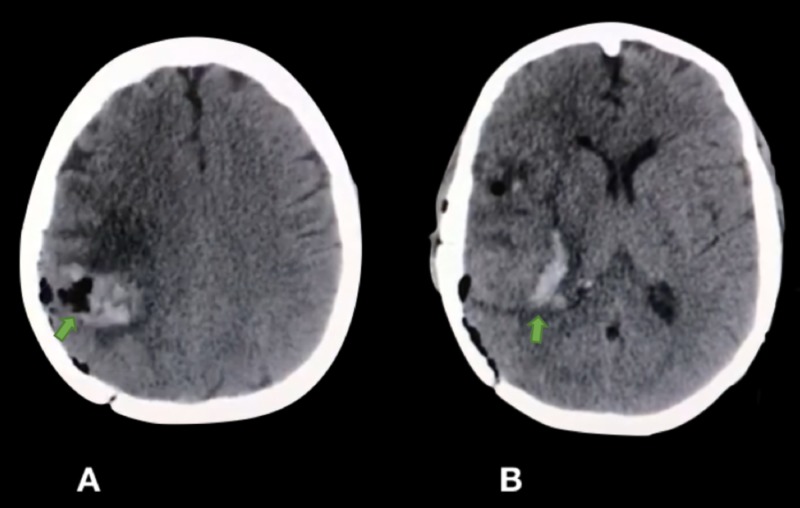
Axial sections of the post-operative computed tomography (CT) scan A: Partial drainage of the hematoma and pneumoencephalon within the lesion; B: Partial drainage of the hematoma.
